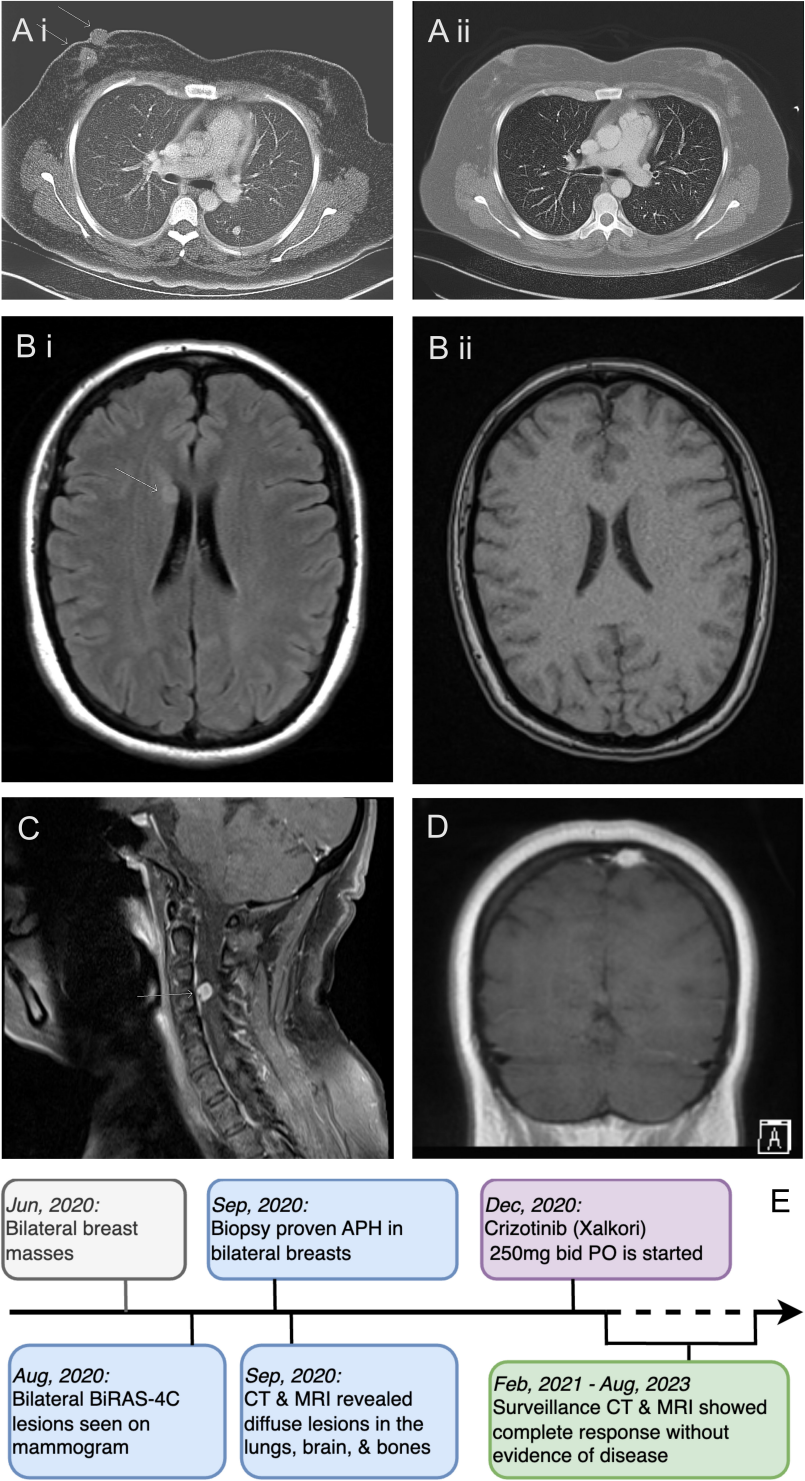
Radiologic features of APH and case timeline. (A) CT scan of the chest with contrast before (A i) and after (A ii) starting crizotinib. Near-complete resolution of the right-side breast mass and left lung nodule are shown. (B) MRI of the brain before (B i) and after (B ii) crizotinib treatment, showing a complete response of the right caudate head lesion. (C) MRI of the spine at initial presentation showing soft disease involvement at the right C3-4 foramen. (D) MRI of the brain showing the calvarial lesion in the posterior left parietal bone at the vertex before treatment. (E) A clinical, diagnostic, and treatment timeline.
